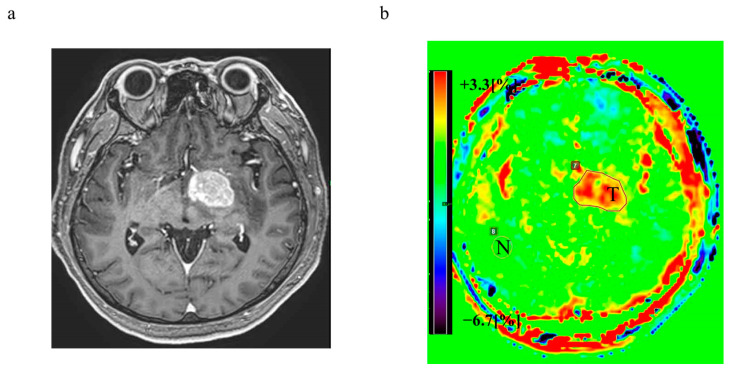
Representative (a) T1-weighted image with gadolinium and (b) ATPw image. T: tumor, N: normal tissue; ATPw image: amide proton transfer-weighted image.
